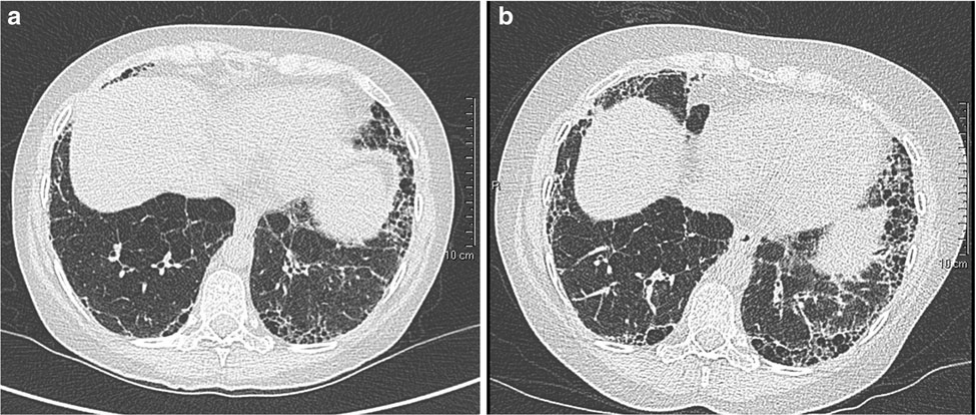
Serial chest HRCT images in relation to IFNα therapy. Progression of subpleural interstitial fibrosis and honeycombing from (a) 9 months prior to the current presentation, prior to commencement of IFNα to (b) 3 months prior, 2 months after commencing IFNα therapy.
